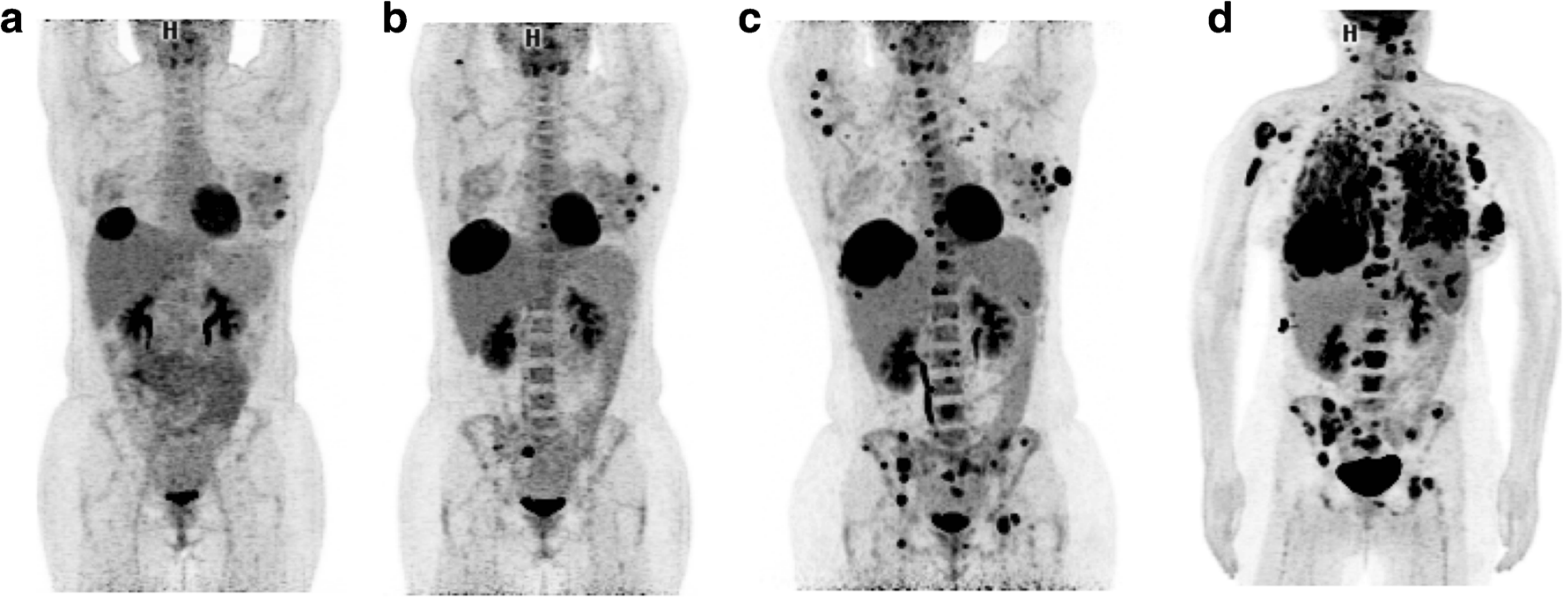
FDG PET images in a melanoma patient with breast and liver metastases treated with nivolumab after progression under anti-BRAF and anti-MEK treatment. a Baseline scan. b Early scan after two cycles shows progression in the breast and liver lesions as well as the appearance of bone metastases. c Scan after six cycles confirms the findings of progression. The case was classified as hyperprogression during immunotherapy (d)
